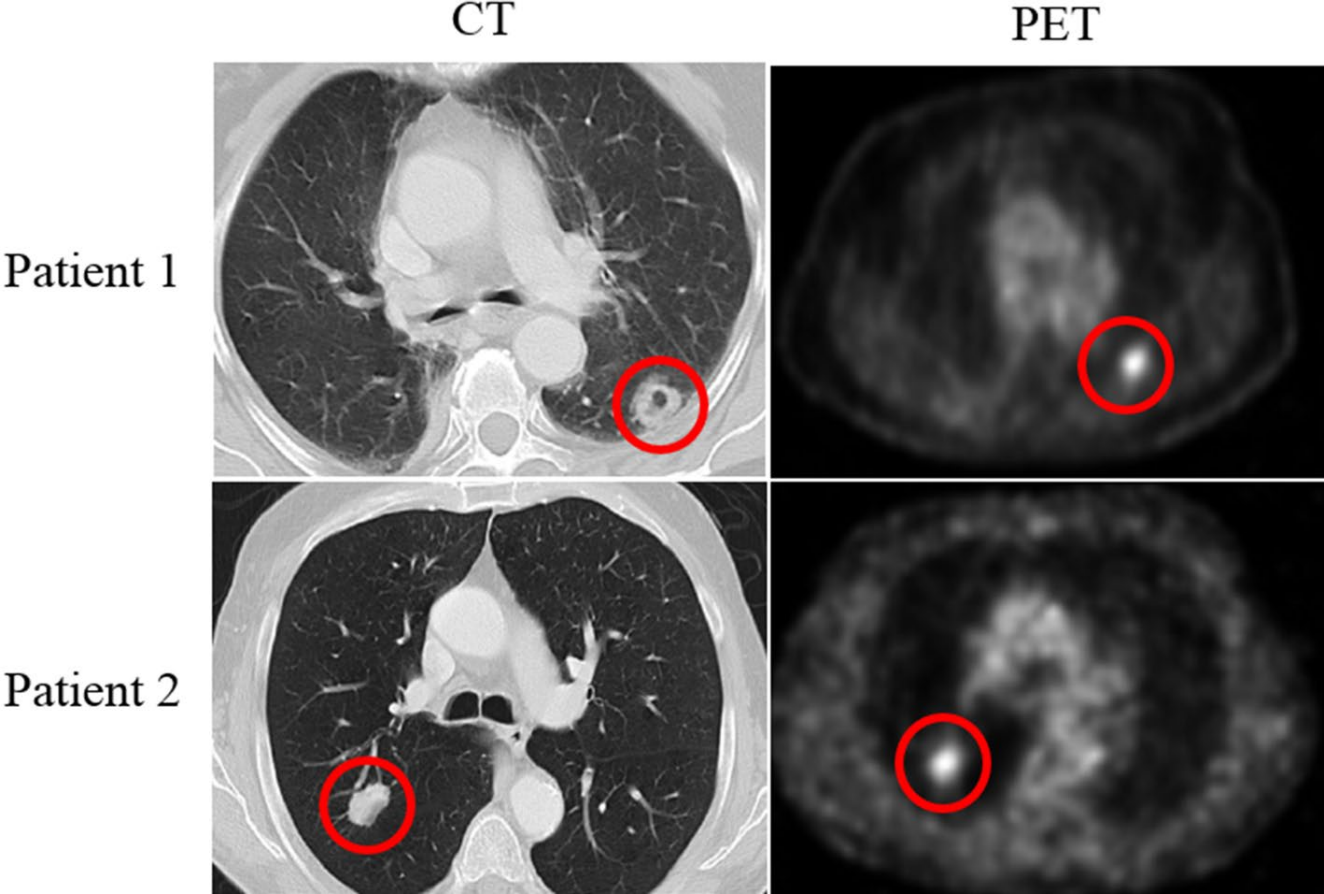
The CT and PET components of the PET/CT, for patient 1 and patient 2, show the tumors in the superior segment of the left lower lobe and the right lower lobe respectively. CT and PET images for each patient are captured at the same level.
